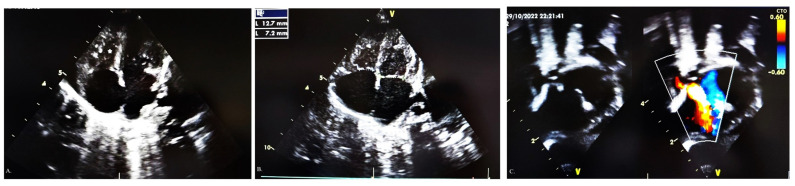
Echocardiographic finding in our patient at the admission: (A) small underdeveloped right ventricle (asterisk); (B) mild hypoplastic tricuspid valve (blue dotted line), 7.2 mm in diameter; (C) right–left shunt on atrial septal defect.
